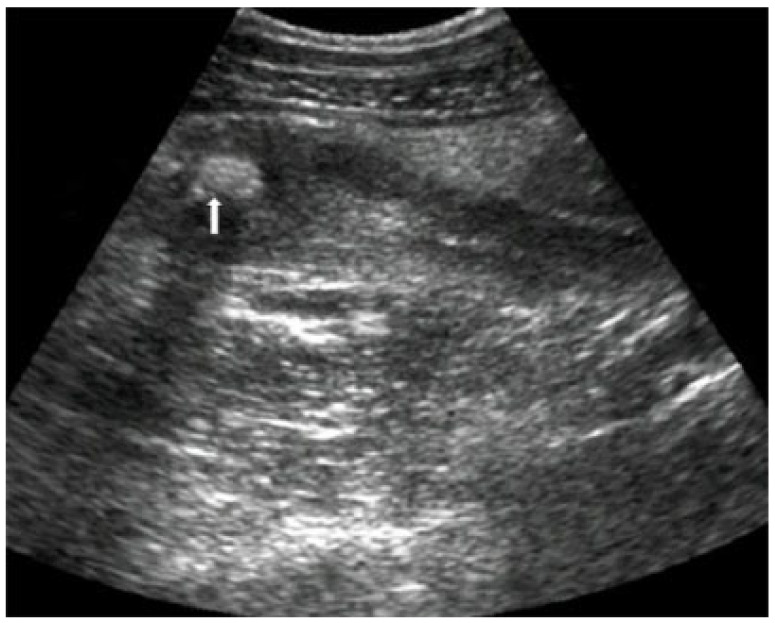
Abdominal ultrasound—Acute appendicitis with visible foreign body (arrow).
